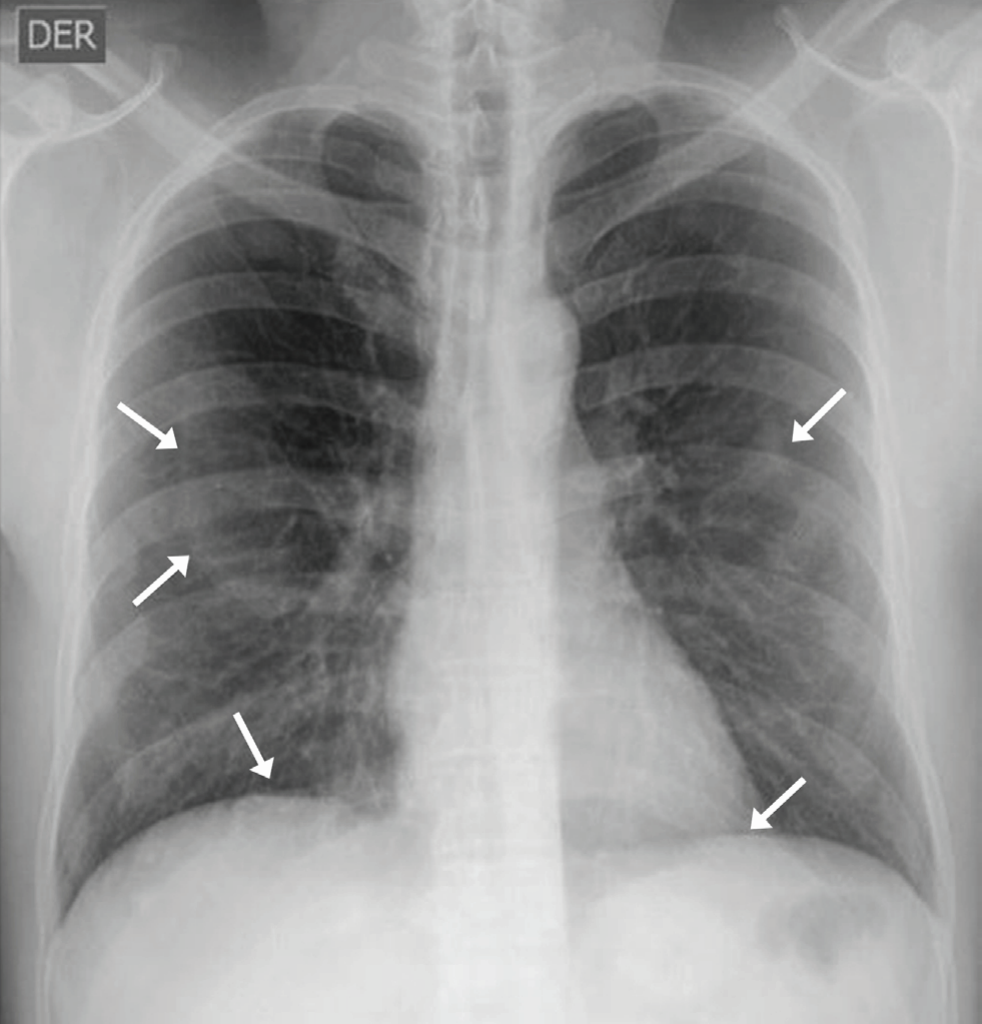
ILO chest x-ray from a patient with history of asbestos fiber exposure showing multiple pleural plaques, some of which presenting with inner calcifications.
